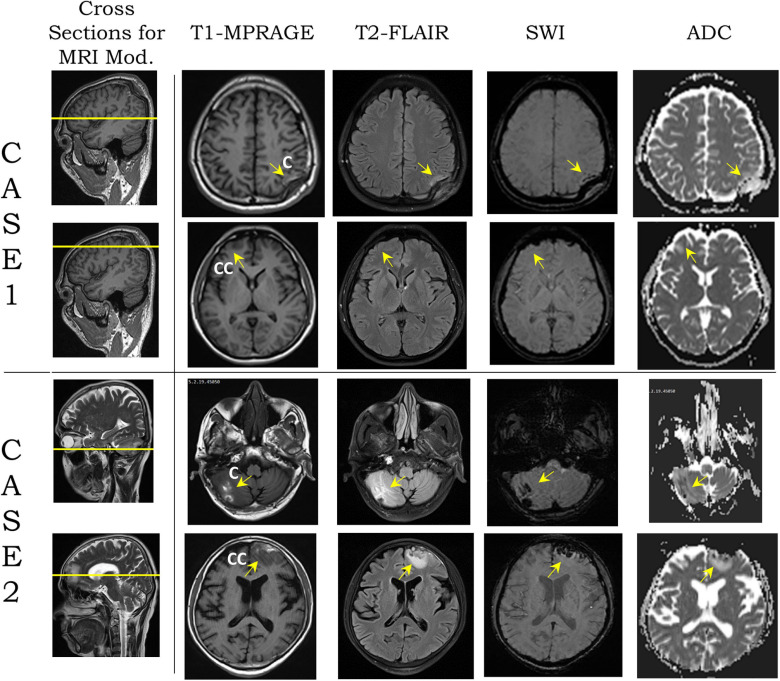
Modalities [T1-magnetization-prepared rapid acquisition gradient echo (MPRAGE), T2-fluid attenuation inversion recovery (FLAIR), susceptibility-weighted imaging (SWI), and apparent diffusion coefficient (ADC)] from magnetic resonance imaging (MRI) images on two transverse planes, shown by yellow lines on sagittal plane section for cases 1 and 2 (second column from left). For each case, the top row shows the coup region images from blunt impact, and the bottom row shows the contrecoup region. Yellow arrows show the coup and contrecoup on modalities. C and CC stand for coup and contrecoup regions, respectively.
